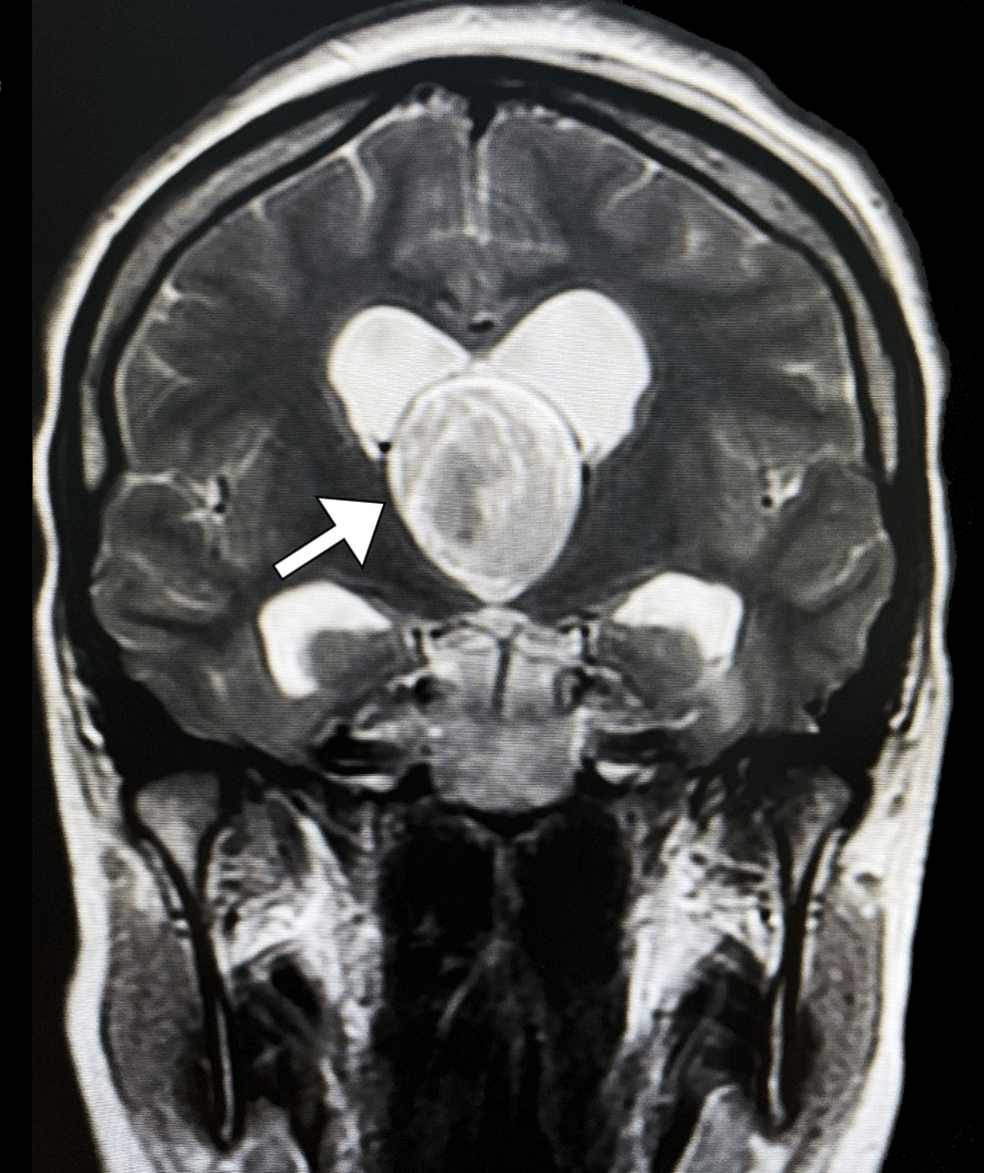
Coronal T2-weighted MRI image of the brain showing a large, heterogeneous mass arising from the roof of the third ventricle, resulting in obstructive hydrocephalus
